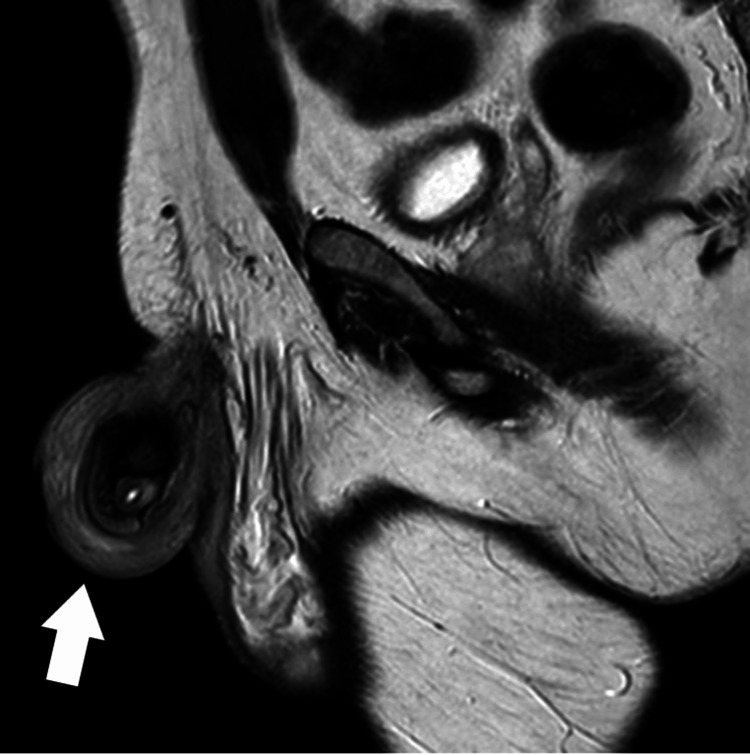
Sagittal plane MRI Image showing soft tissue swelling
